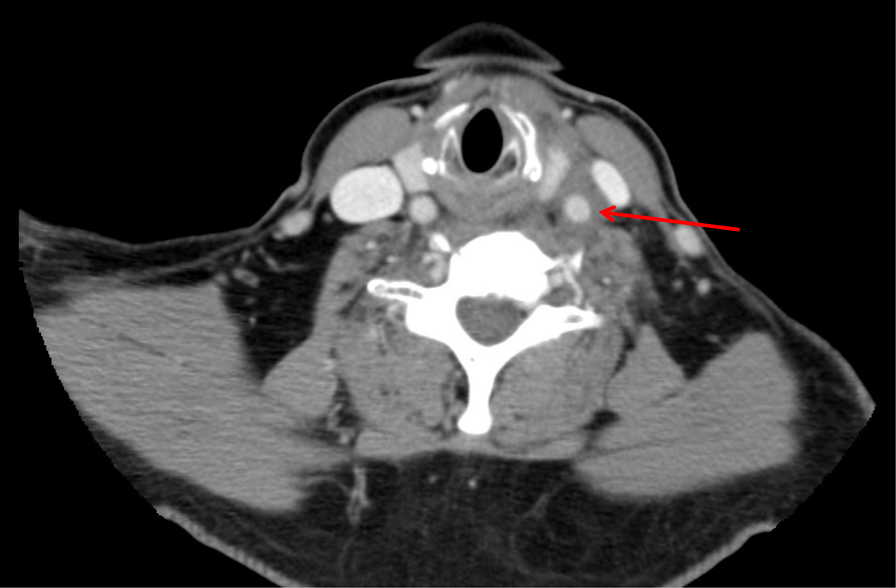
Computed tomography of the neck on initial presentation revealing wall thickening of the left distal common carotid artery with hazing of the adjacent fat (*red arrow*)
